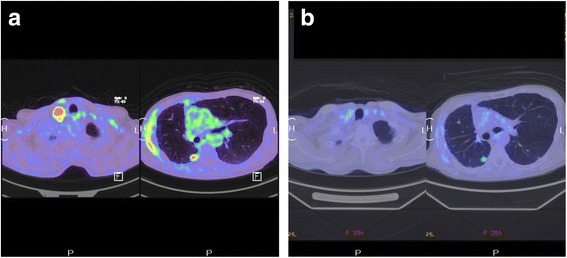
FDG uptake before (a) and after (b) immunotherapy. Maximum standardized uptake value (SUVmax) of supraclavicular lymph node and disseminated lesions before and after immunotherapy decreased from 9.8 to no accumulation and 5.9 to 3.4, respectively. Each SUVmax was decreased after six courses of nivolumab treatment
